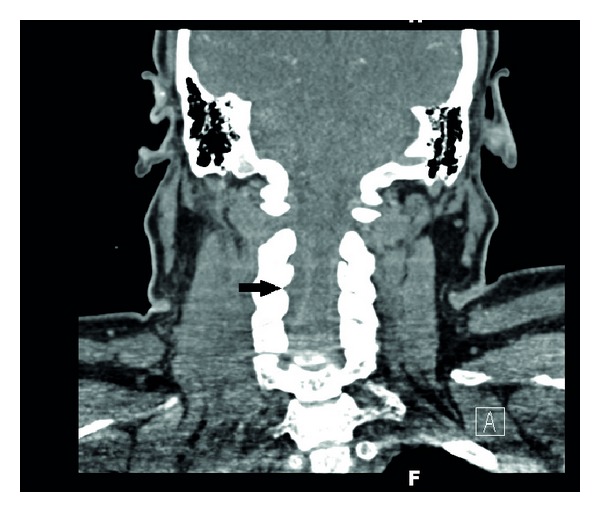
CT of the neck revealing a 3.7 by 1.4 cm low-density oval lesion (arrow) displacing the thecal sac and extending from the C2 to the C4 vertebrae.
